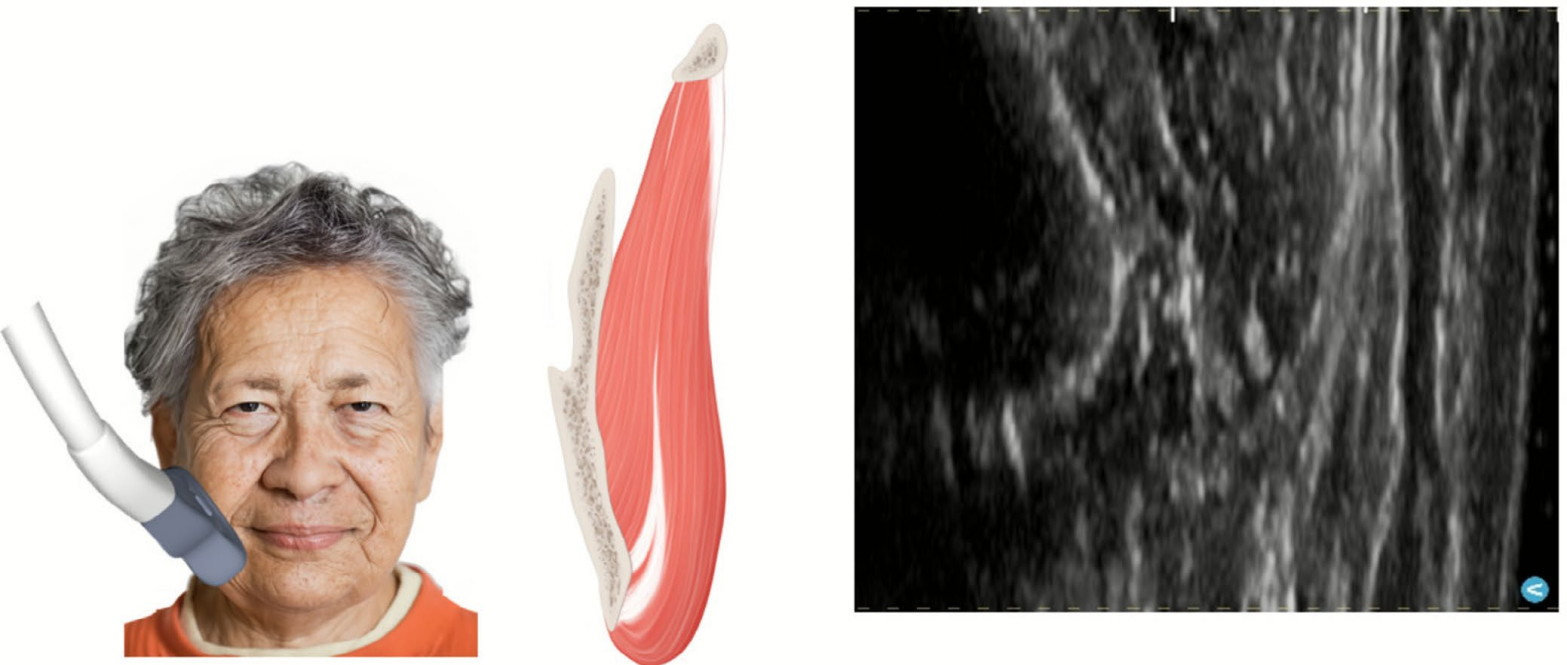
Ultrasound imaging reveals inferior displacement of the masseter muscle beyond the mandibular border in an elderly patient, demonstrating loss of gonial angle definition. The sagging muscle fibers and progressive thinning contribute to submandibular contour changes, challenging the traditional attribution to fat redistribution alone.
